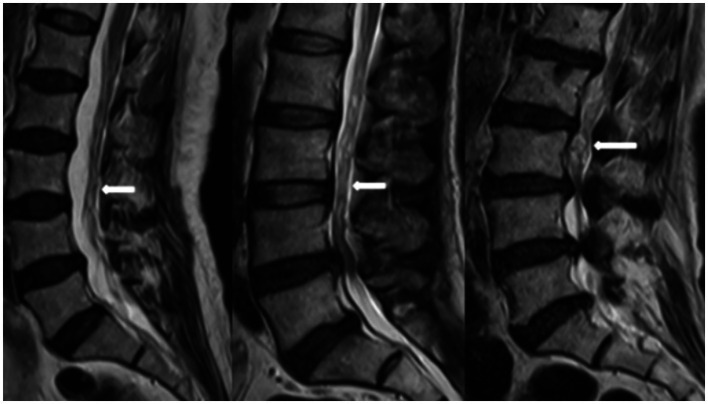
RNRs show thickening, buckling, and serpentine- or loop-shaped on their T2-weighted sagittal MRI slices.
